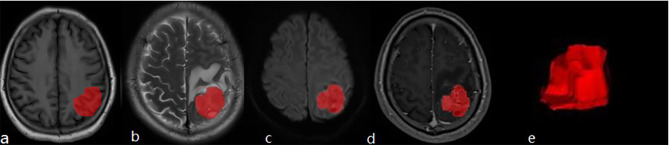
(a-d) The red areas showed the tumor level of T1WI, T2WI, DWI, T1-enhanced, (e) the generated tumor 3D-VOI
